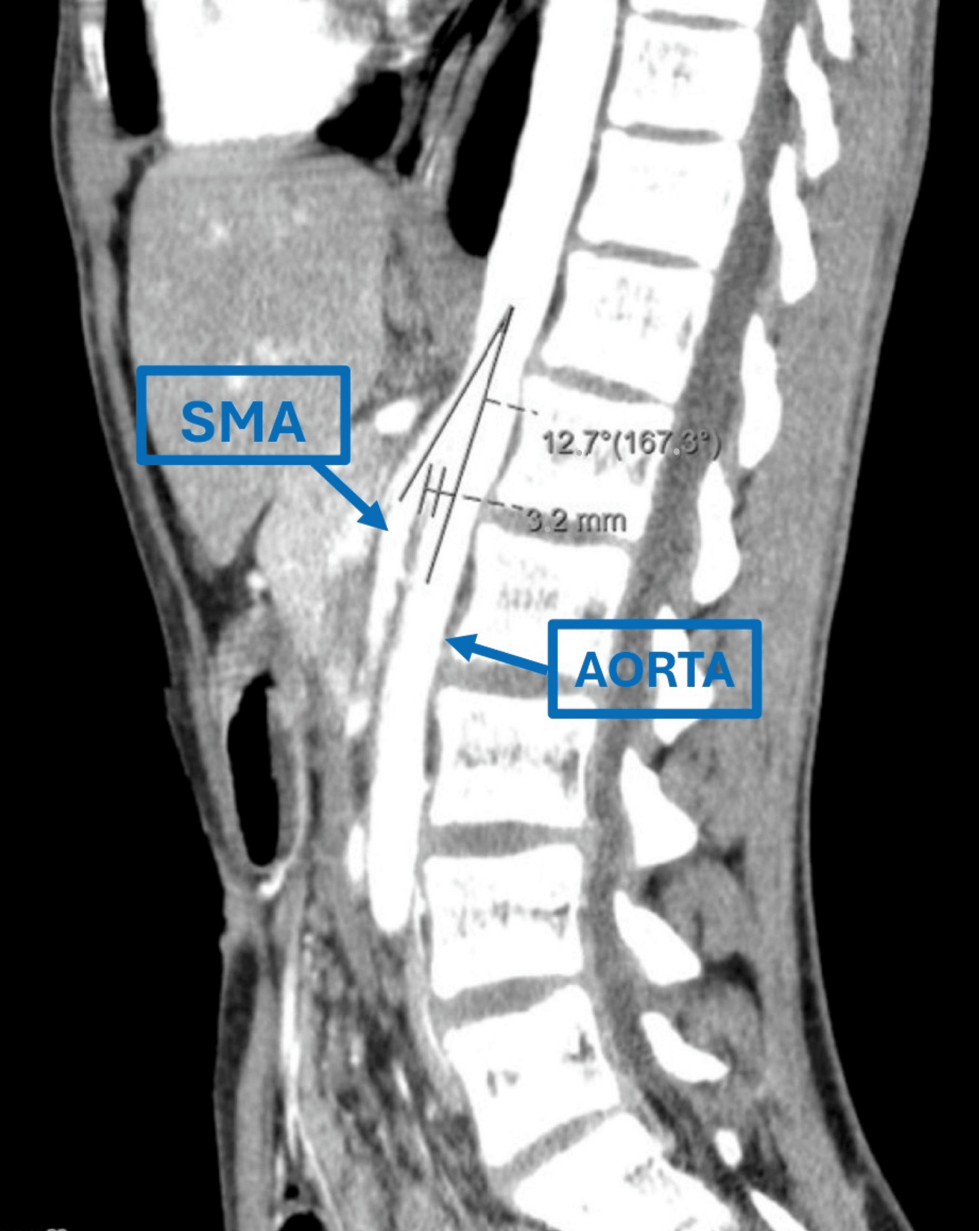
Sagittal Plane Computed Tomography (CT) Scan Sagittal plane abdominal CT scan showing superior mesenteric artery (SMA) compression of the duodenum. The aortomesenteric angle is reduced to 12.7° and the aortomesenteric distance narrowed to 3.2 mm, findings consistent with superior mesenteric artery syndrome (SMAS).
